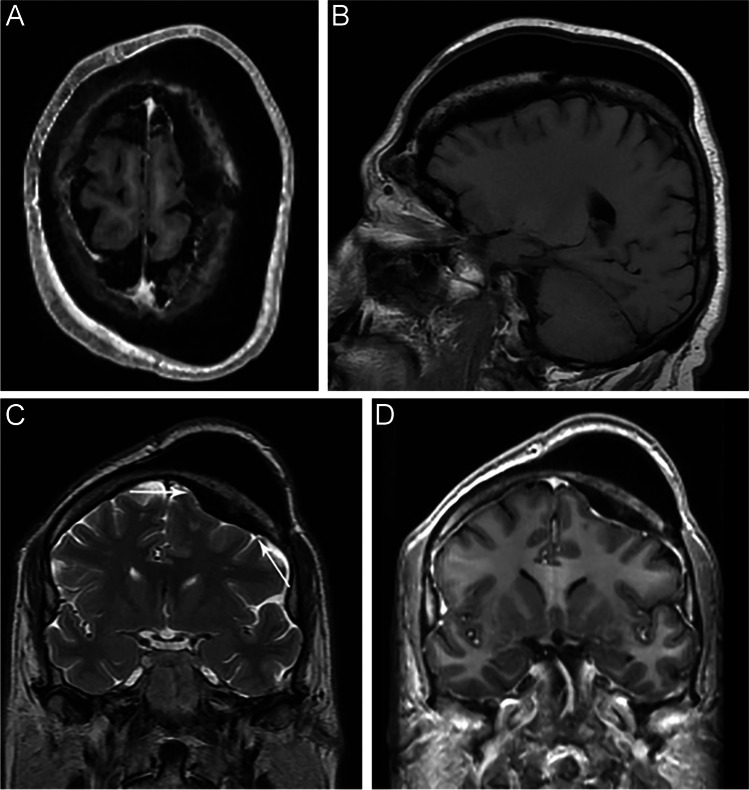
An MRI brain obtained 8 years earlier. Axial (A) and sagittal (B) T1 weighted turbo spin echo (TSE), coronal T2 weighted sequence (C), and coronal gadolinium-enhanced T1 weighted sequence (D). The massive cortical thickening has not significantly changed in appearance in the 8 years since this study was performed. As it is cortical bone, it appears black on all sequences, and there is no evidence of contrast enhancement
